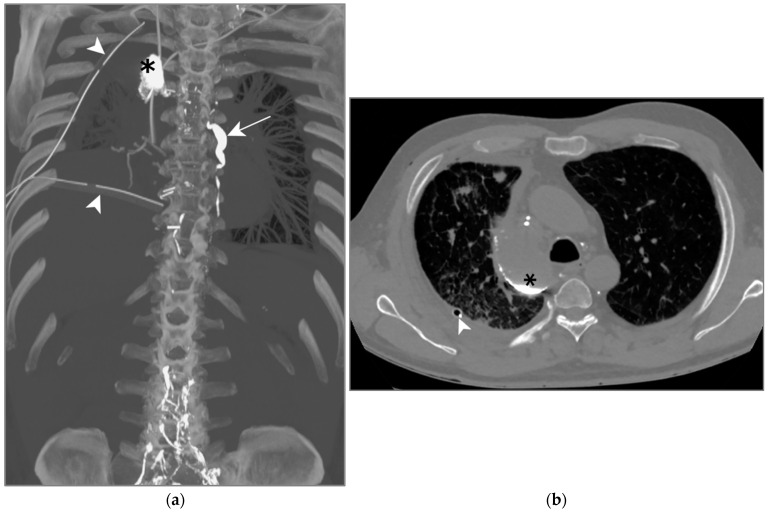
Patient C. The maximal-intensity projection of the CT scan reconstructed in the coronal plane (a) and the axial CT plane through the upper pulmonary lobes (b). (a,b) Accumulation of hyperattenuating Lipiodol in an encapsulated collection in the apical portion of the right pleural space (black asterisks). The ductus thoracicus is dilatated (white arrow). Two right pleural catheters are shown (white arrowheads).
